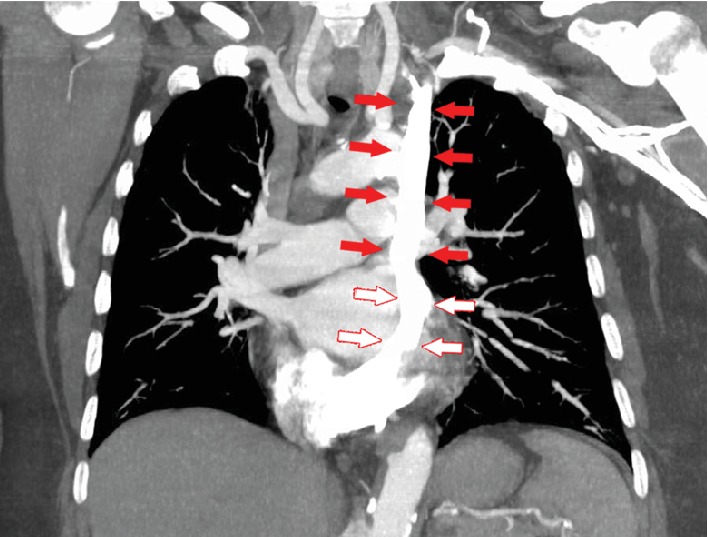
CT angiogram chest that demonstrates duplication of the superior vena cava (red arrows) with a persistent left superior vena cava, which drains into the coronary sinus (red outline arrows) and then the right atrium.
